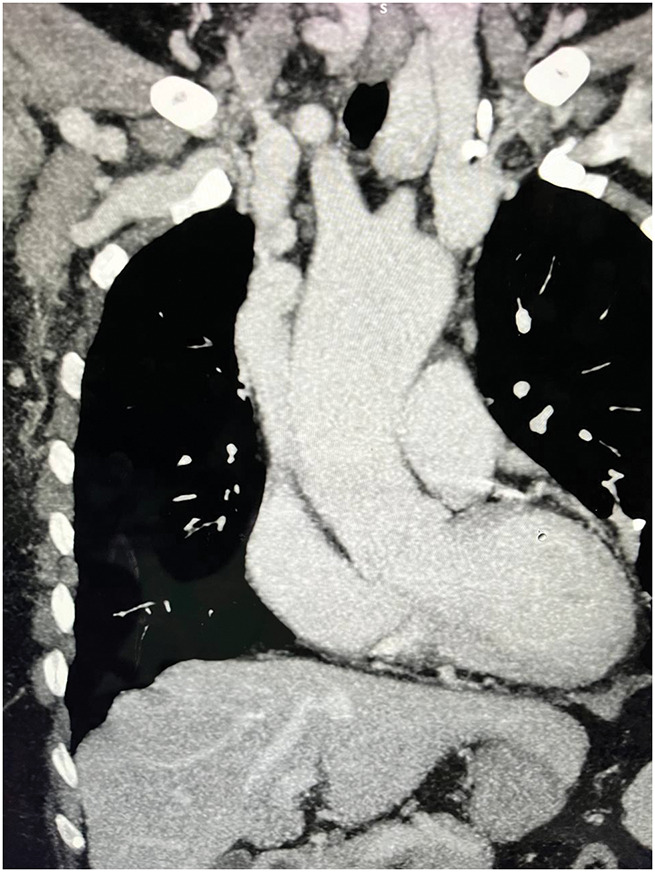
Venous-phase contrast-enhanced CTA demonstrating CTO of the right brachiocephalic vein. The right brachiocephalic vein is not opacified on venous-phase imaging, consistent with complete fibrotic occlusion. The superior vena cava and left brachiocephalic vein demonstrate normal contrast enhancement. Extensive mediastinal collateral formation is present, indicative of long-standing central venous occlusion. CTO: chronic total occlusion; CTA: computed tomography angiography
